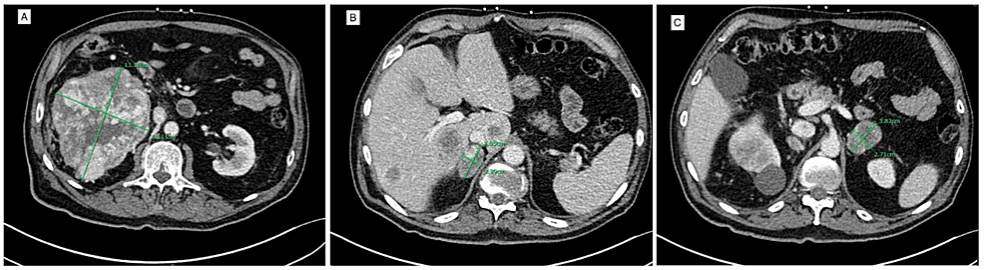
(A) Axial view of the abdominal CT scan demonstrating a heterogeneous hyperenhancing mass in the right kidney measuring 10.9 x 13.1 x 11.4 cm, diagnosed as renal cell carcinoma. (B) Heterogeneous enhancing mass in the right adrenal gland measuring 3.9 x 2.4 cm. (C) Heterogeneous enhancing mass in the left adrenal gland measuring 3.8 x 2.7 cm.
